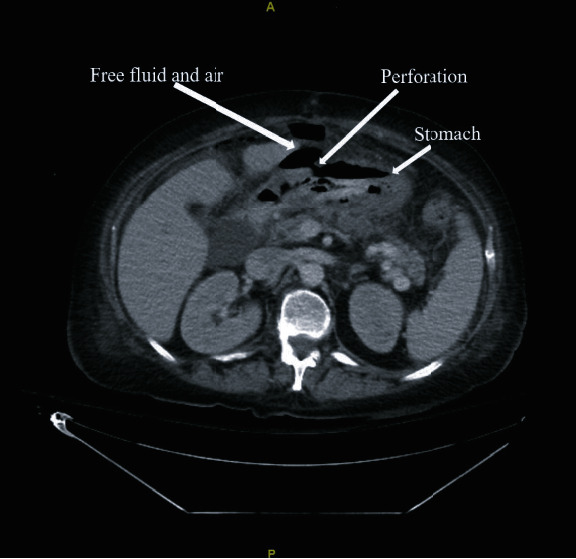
Patient 2 CT abdomen exhibiting bowel perforation secondary to mucormycosis.
